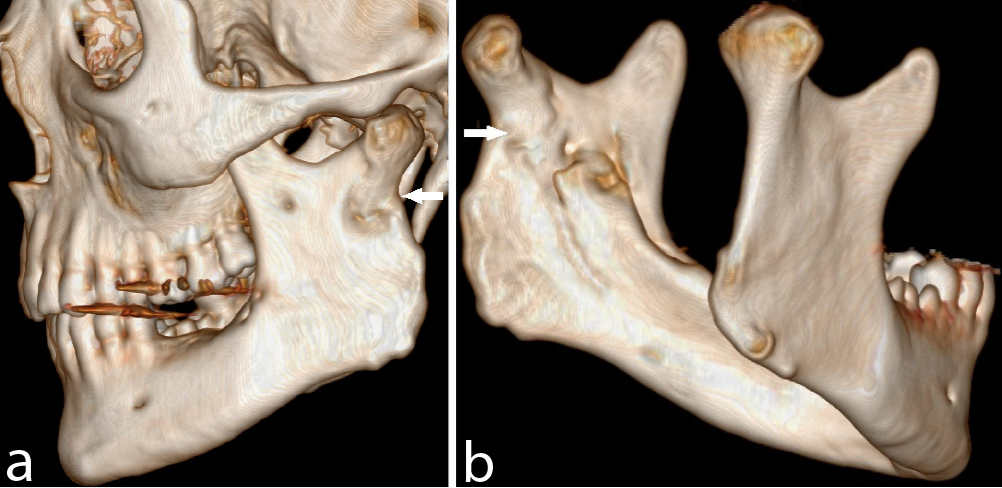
Six-months postoperative 3D-CT volume-rendered reconstructed images demonstrating healing of the condylar fracture in a slightly shortened and anteriorly displaced position. Arrows mark the healed fracture.
